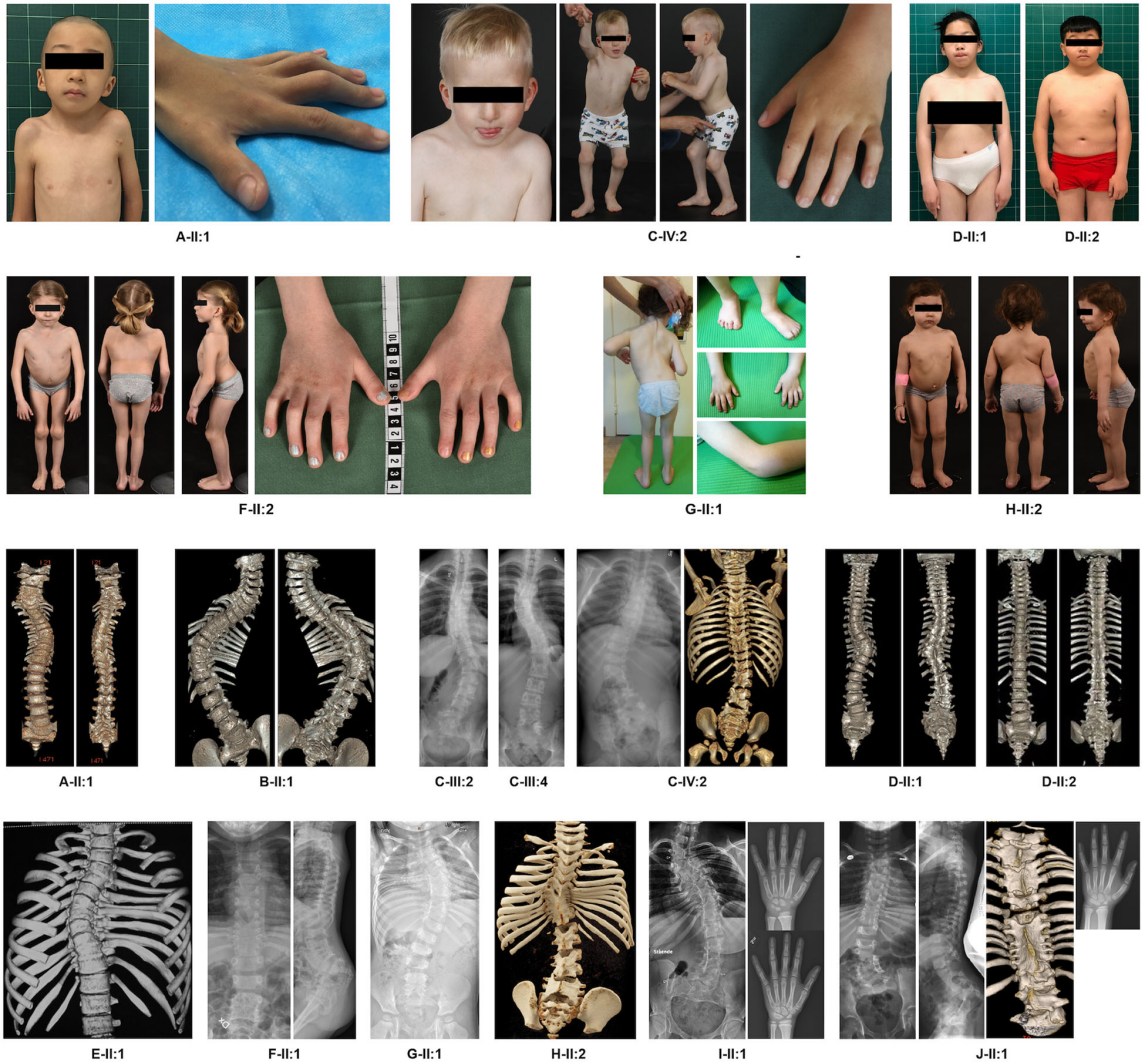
Phenotypic and radiographic features of the patients with pathogenic *MYH3* variants. Please note the following features: A-II:1 has small mouth with dowslanting corners, low-set ears, prominent nasal bridge and camptodactyly. C-IV:2 has small mouth, broad chest, increased distance between the nipples, enlarged knee joints with pterygia, flat feet and camptodactyly. D-II:1 has small mouth with downslanting corners, small low set ears, prominent philtrum and increased distance between the nipples. D-II:2 has small mouth with downslanting corners, small low set ears, prominent philtrum and ptosis. F-II:2 has broad chest, scoliosis, enlarged knee joints, elbow and knee contractures and bilateral clinodactyly of the 5th finger. G-II:1 has scoliosis and pterygia. H-II:2 has broad chest, scoliosis and mild pterygia of the knee. Radiographic features: A-II:1 has multiple cervical and thoracic vertebral fusions, rudimentary disc spaces and scoliosis. B-II:1 has multiple, mainly thoracic, vertebral fusions with rudimentary disc spaces, rib crowding and scoliosis. C-III:2 and C-III:4 both have moderate scoliosis and a few thoracic and lumbar/lumbosacral fusions. C-IV:2 has multiple posterior fusions of the cervical, thoracic and lumbar spine and scoliosis. D-II:1 has several thoracic and lumbosacral fusions with a distinct curve due to asymmetric T9 vertebral body. D-II:2 has some posterior vertebral fusions, mostly thoracic, without scoliosis. E-II:1 has multiple thoracic vertebral fusions, mild rib crowding and scoliosis. F-II:2 has multiple thoracic and lumbar vertebral fusions with rudimentary disc spaces, rib crowding and marked lumbosacral lordosis without scoliosis. G-II:1 has multiple thoracic vertebral fusions, rib crowding and scoliosis. H-II:2 has multiple thoracic and lumbar/lumbosacral posterior fusions, rib crowding and scoliosis. I-II:1 has multiple thoracic and lumbar vertebral fusions, rib crowding and scoliosis. Hand radiograms of I-II:1 at 5 and 8 years of age show development of lunotriquetral fusion. J-II:1 has multiple thoracic and lumbar vertebral fusions, rib crowding and scoliosis at 4 years 4 months of age. Hand radiogram shows lunotriquetral fusion.
